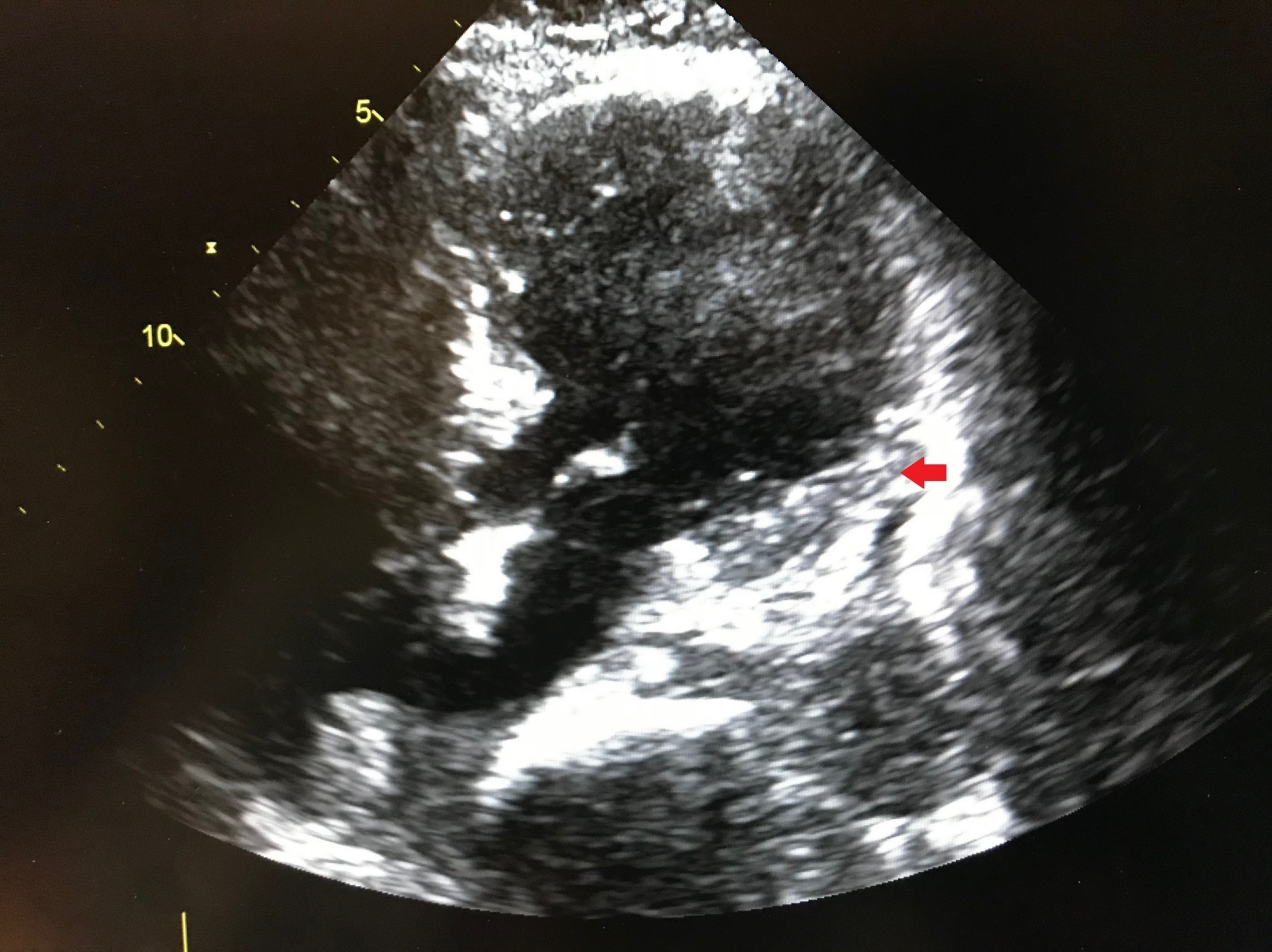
Echocardiogram (apical 2 chamber view) showing systole with apical ballooning of the left ventricle (arrow).
